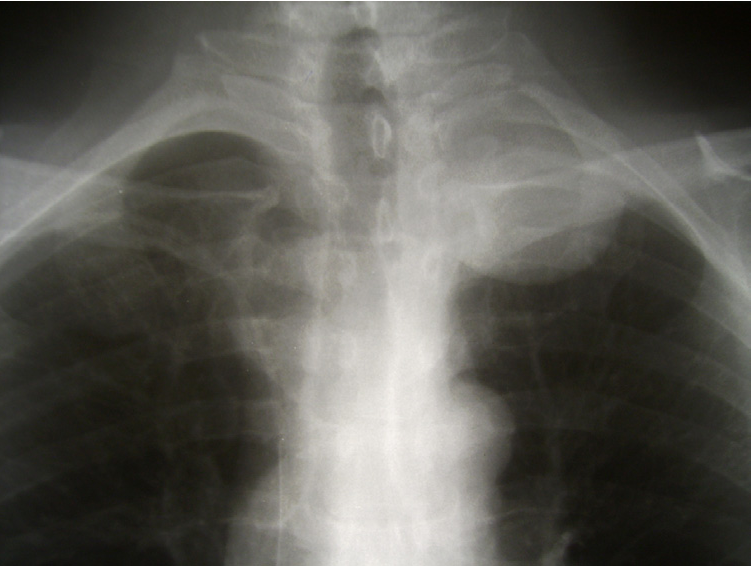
Pre-operative chest X-ray demonstrating a round shadow of approx. 5 cm in diameter in the left pulmonary apex.
